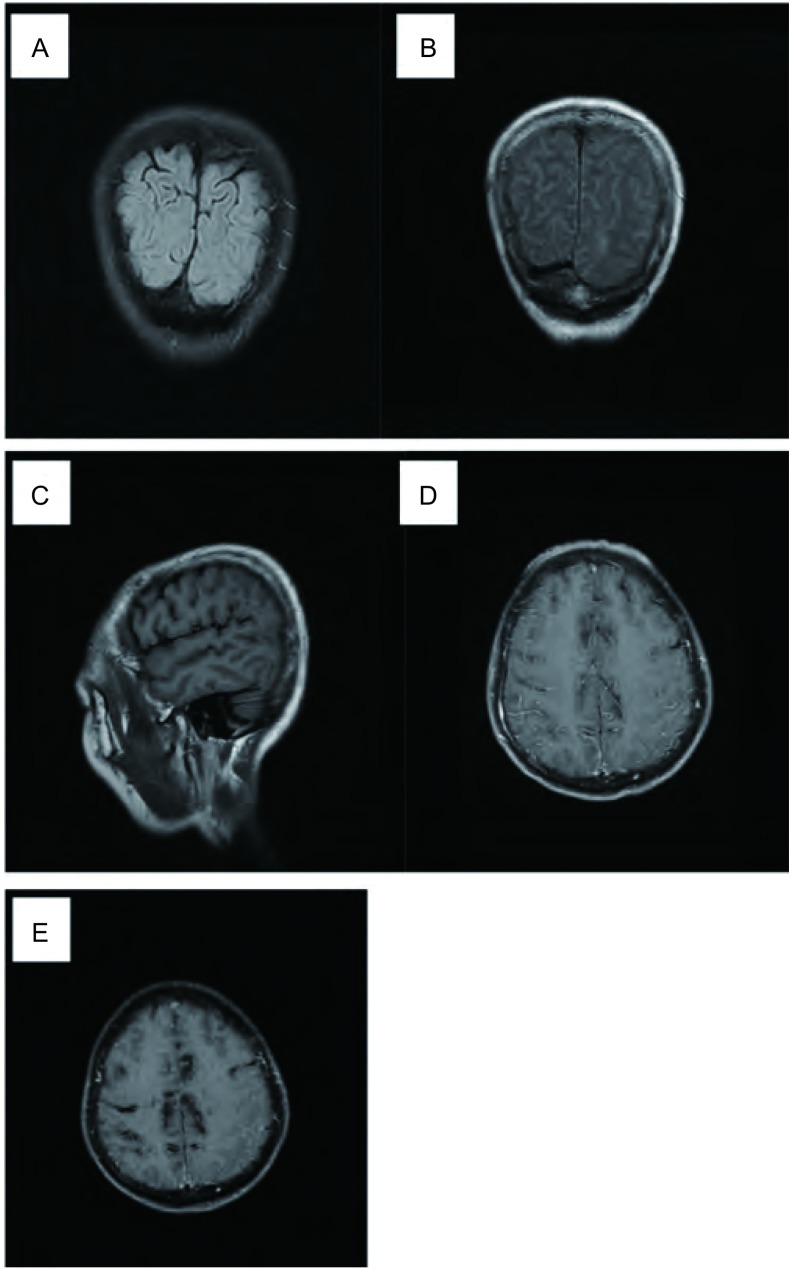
脑MRI改变。A:2015-01-12 T2FLAIR序列未见明确转移灶; B and C:2015-05-17治疗前; MRI:T2FLAIR相冠状位及T1WI矢状位图像与A图像比较, 双侧顶叶脑沟变窄; D:2015-05-17治疗前; MRI:轴位T1WI增强序列显示双侧额顶叶沿脑沟分布的线状明显强化影; 右侧软脑膜增厚并明显强化; E:2015-09-18治疗后; MRI:轴位T1WI增强序列显示, 双侧额顶叶脑沟内线状强化影有所减少。 Patterns of brain MRI.A:No metastases in the T2FLAIR image; B and C:2015-05-17;MRI:before treatment:The coronal T2FLAIR and sagittal T1WI image demonstrated shallow sulcus in bilateral parietal lobe compared with image A; D:2015-05-17;MRI:before treatment:The axial T1WI enhanced image showed linear enhancing meninges along the surface of bilateral frontal and parietal lobe; meninges was thicking and enhancing on the right side; E:2015-09-18 MRI after treatment:The axial T1WI enhanced image showed decreased along the surface of bilateral frontal and parietal lobe.
